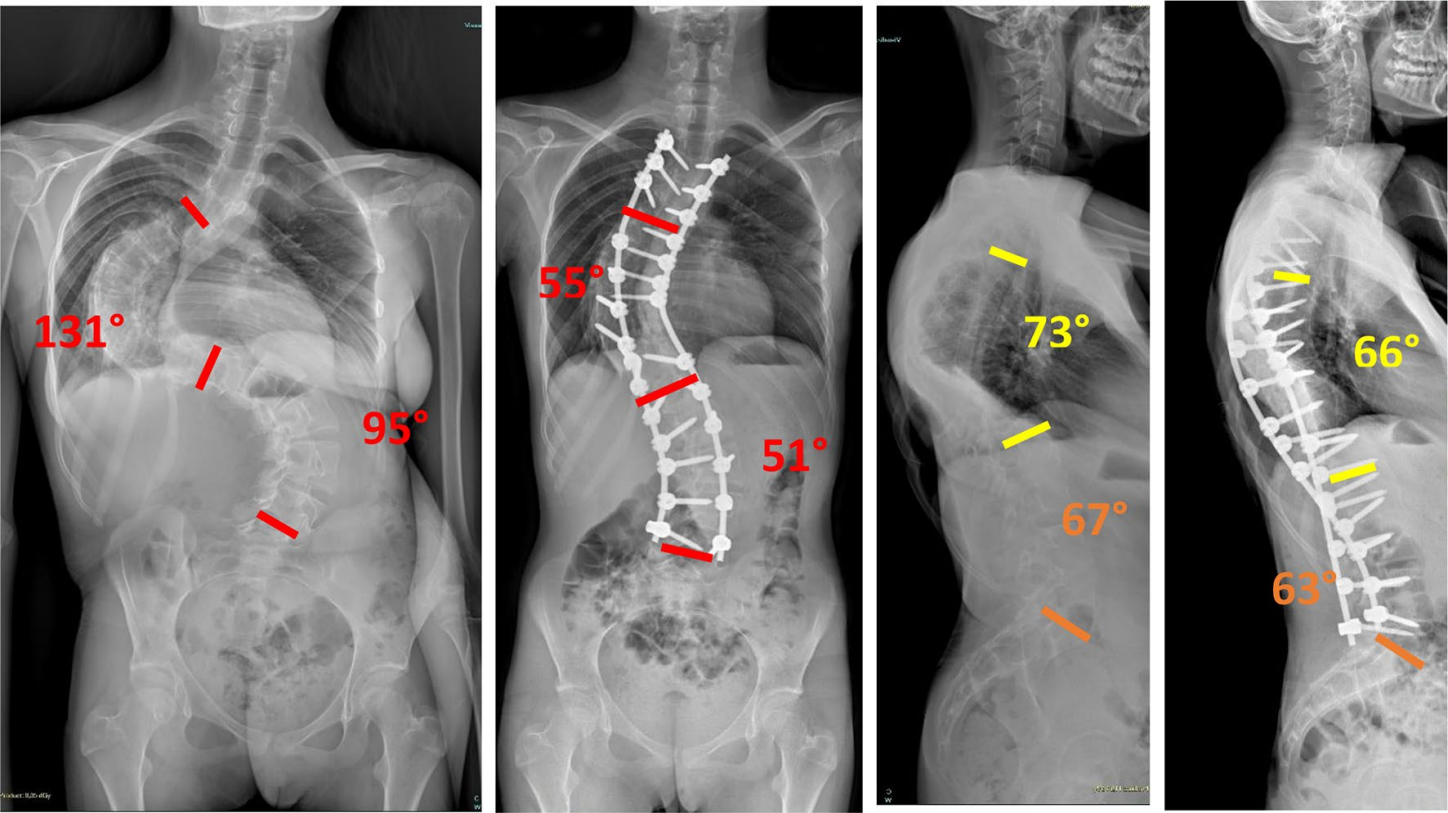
Case 3, a 13 years old female with a Lenke 3 curve, treated with a T3–L5 fusion; pre- and post-operative X-rays
